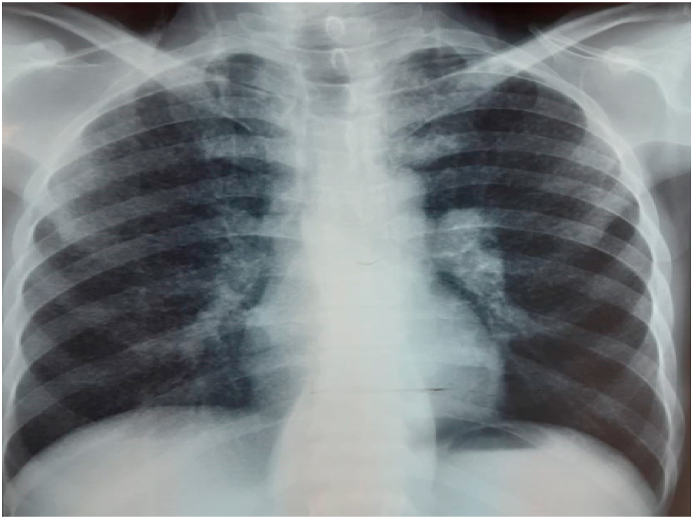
Chest X-ray of our patient: diffuse micronodular opacities in both lung fields giving a Milliary appearance.
